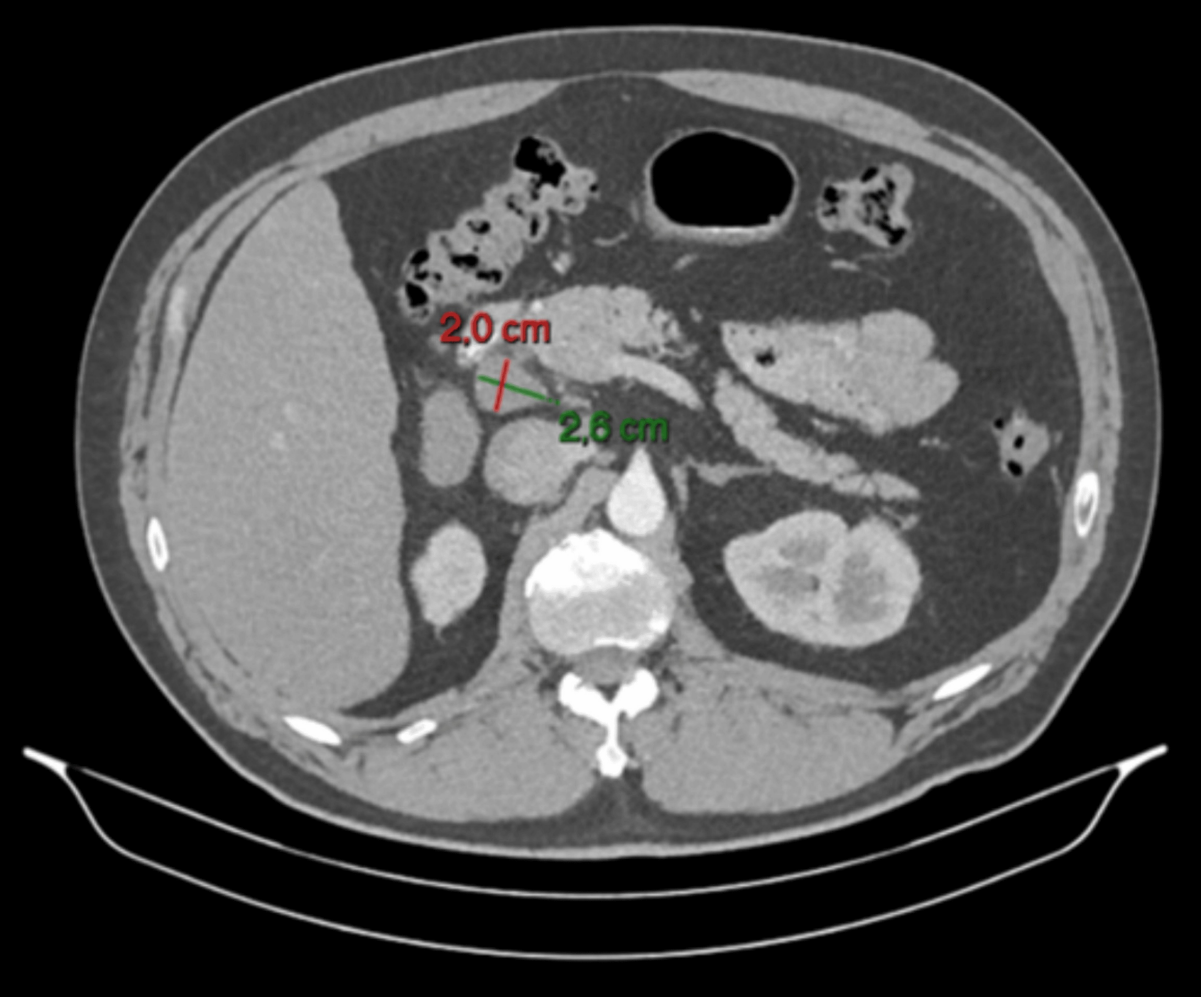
Follow-up CT abdomen (axial view), showing persistent lymph node adjacent to the pancreatic head (2.6 × 2.0 cm), unchanged in size
